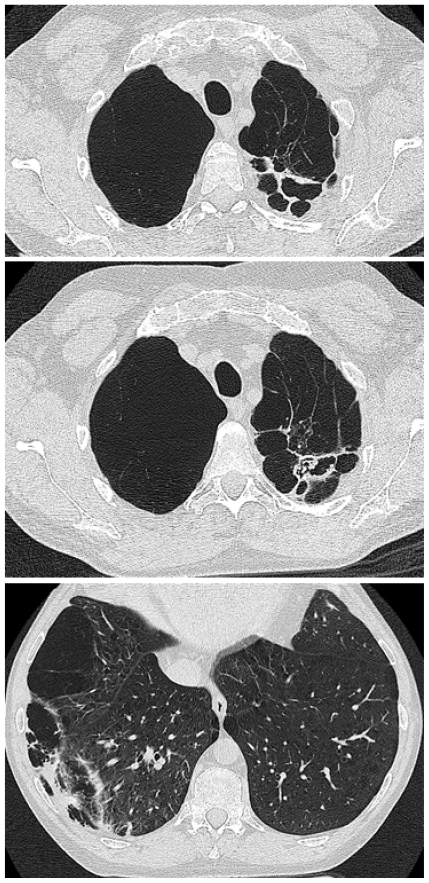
Patient 2 CT scan cuts of the thorax showing extensive bullae formation on the right, cavitation posteriorly on the left, with fungal material present in a cavity on the second section, and additional areas of cavitation in the right lower lobe, with slightly thicker walls and pleural involvement.
